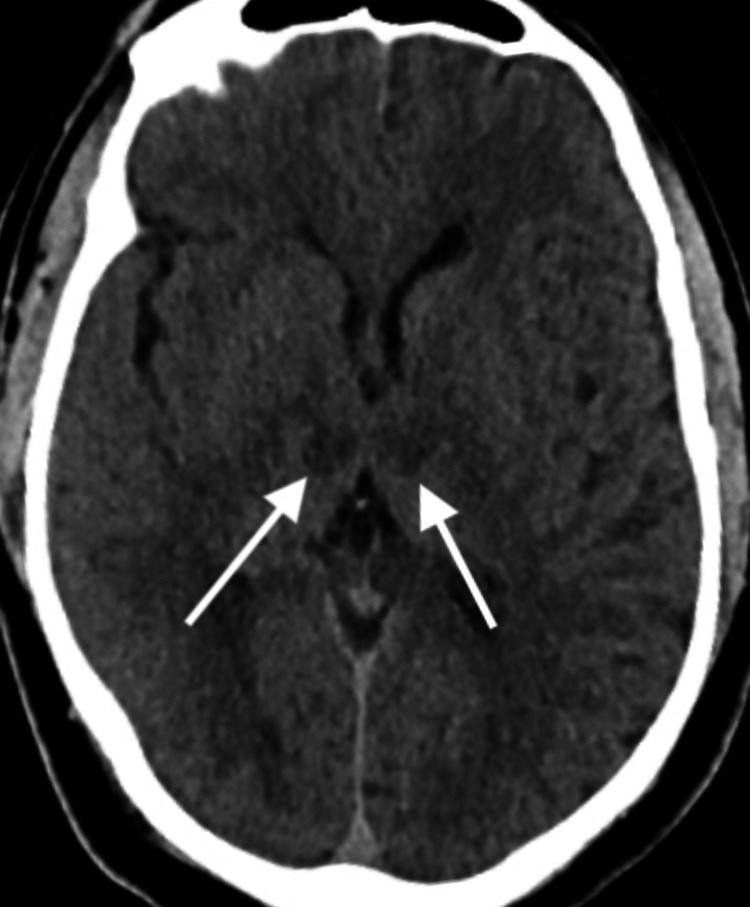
Computed tomography of the head, axial view, showing bilateral symmetric thalamic hypodensity.
